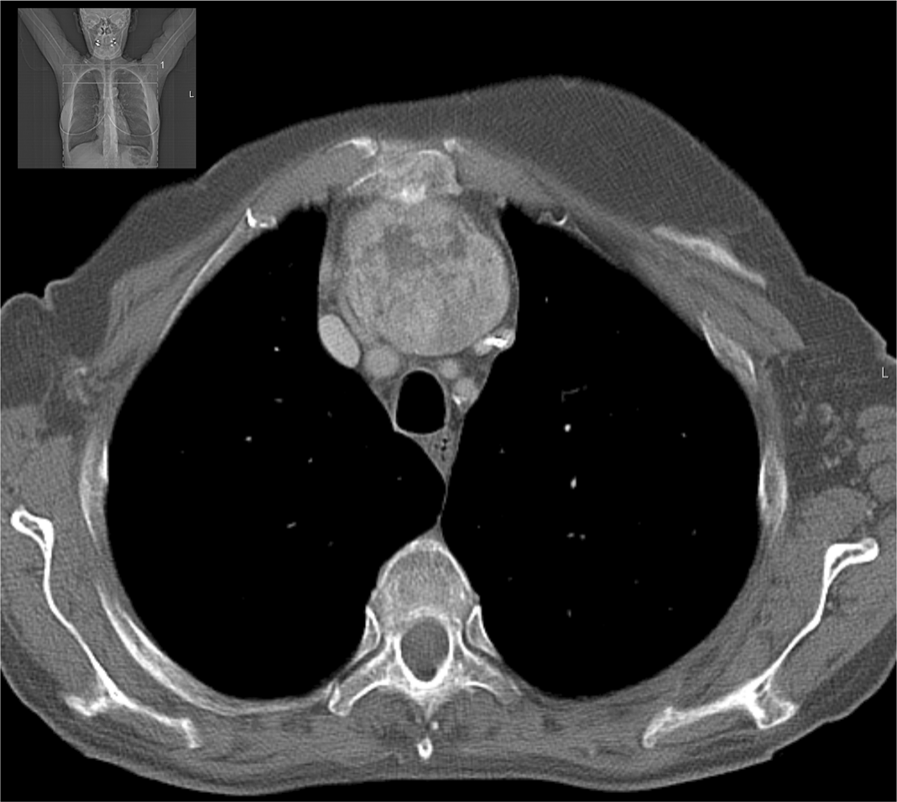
Neck and thorax computed tomography scan showing the heterogeneous 67 × 46 × 52 mm mass in the anterior mediastinum – axial section
